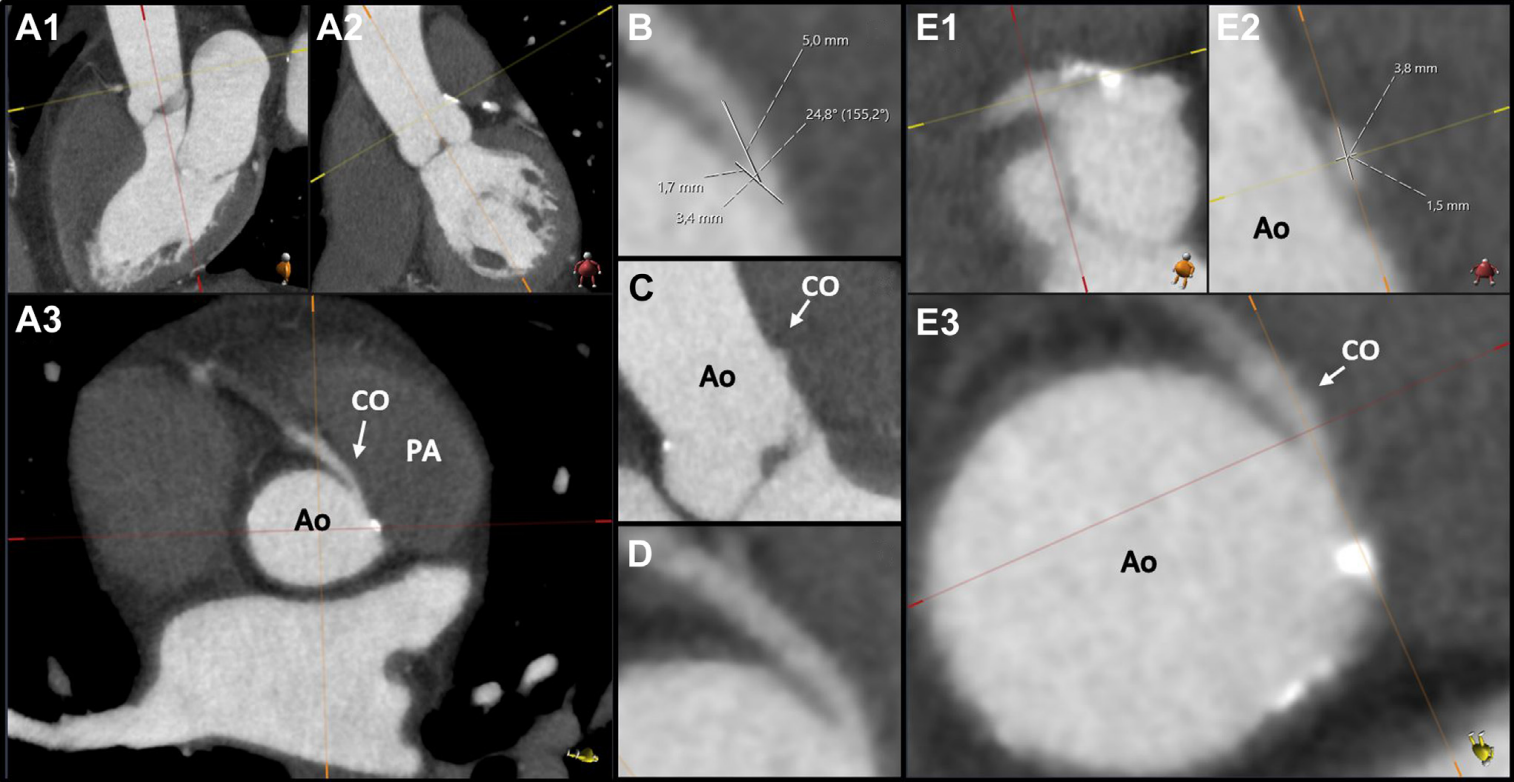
CTA analysis approach. (A_1_-A_3_) Three-dimensional multiplanar reconstruction with double oblique planes perpendicular to the aortic annulus. (B) Acute-angle take-off measurement. (C) Orifice take-off in relation to the sinotubular junction. (D) Assessment of an intramural course and its length. (E_1_-E_3_) Orifice shape measurement. Ao, aorta; CTA, computed tomography angiography; CO, coronary ostium of the AAORCA; PA, pulmonary artery.
